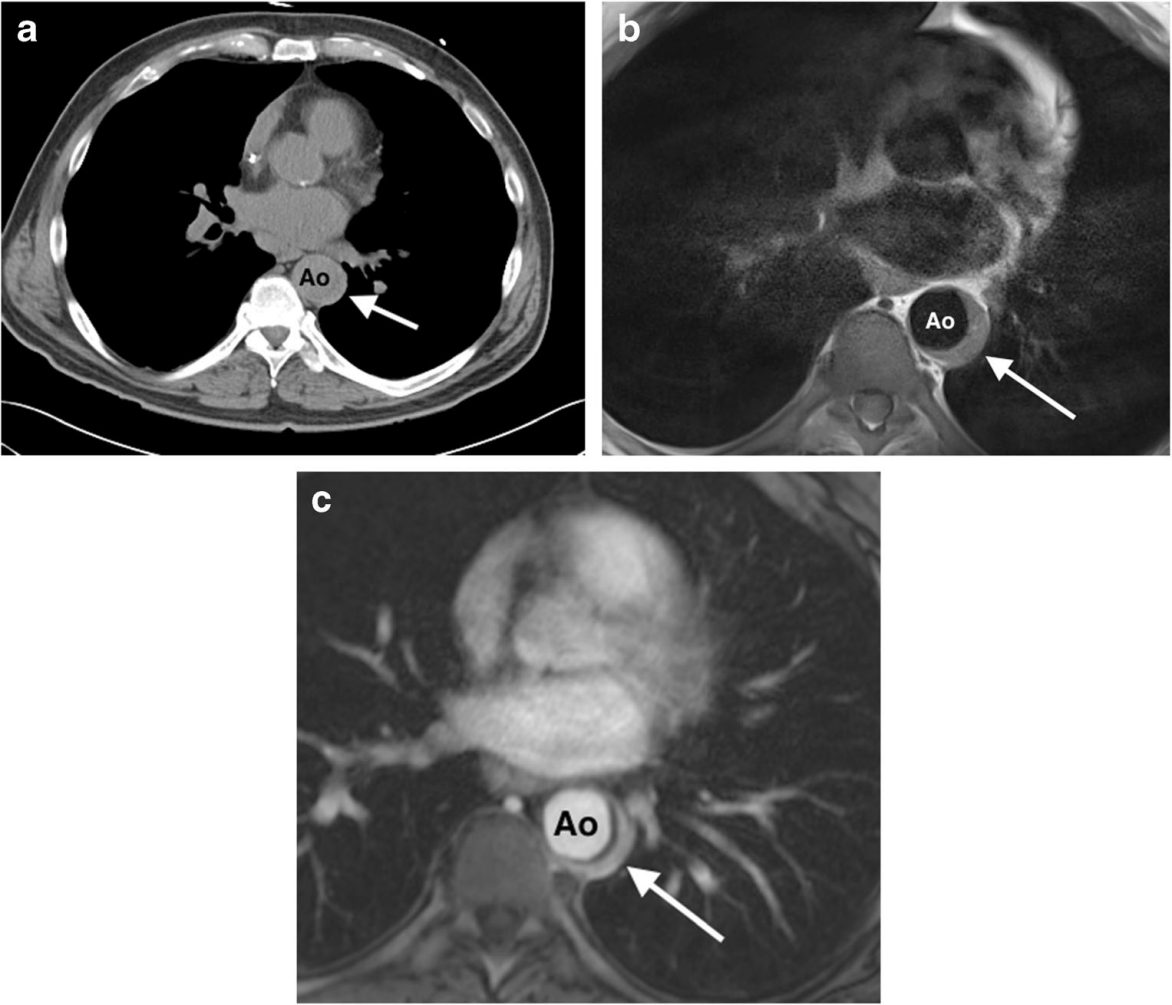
A 55-year-old man with a history of chronic myeloid leukemia presents with fever and chest pain. (a) An axial non-contrast CT scan of the thoracic aorta demonstrates crescentic mural thickening (*arrow*) of the mid-descending thoracic aorta. (b) An axial T1 double inversion recovery (DIR) MRI pre-contrast image demonstrates crescentic mural high signal in the same location in the descending thoracic aorta. (c) An axial T1 MRI post-GBCA image demonstrates crescentic mural enhancement in the corresponding location, consistent with an aortitis. The patient had new cryptococcal sepsis, with no other focus of infection, and a diagnosis of cryptococcal aortitis was made. The patient died despite systemic antifungal treatment. *Ao = descending thoracic aorta*
